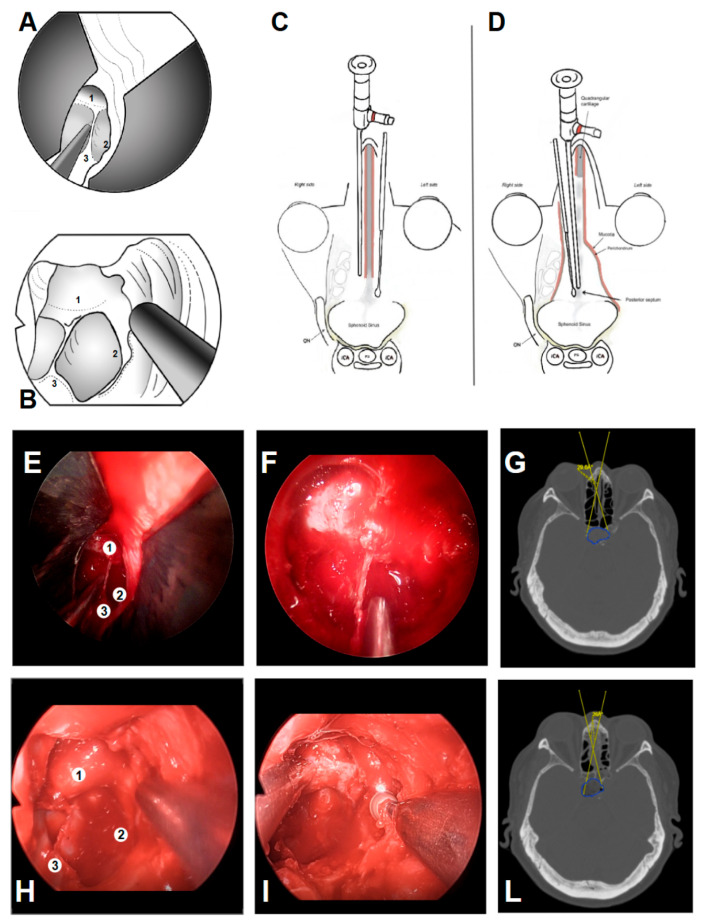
Comparison of the EONOTTA (A) and binostril EEA (B). EONOTTA (E,F) and binostril EEA (H,I) initial and deep endoscopic views showing the initially narrow entry corridor, with similar deep visualization of landmarks such as the sellar bulge (1), posterior sphenoid sinus wall (2), and septum (3). (C,D) Pre-operative CT scans demonstrating the expected working angles and the corresponding tumor regions for the EONOTTA patient ((G): 29.6°) and the binostril EEA ((L): 29.7°).
